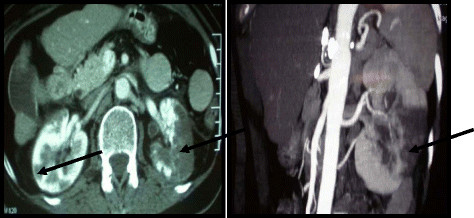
Axial and Coronal enhanced CT angiography: parenchymal perfusion defects of both kidneys related to segmental distal arterial lesions.
